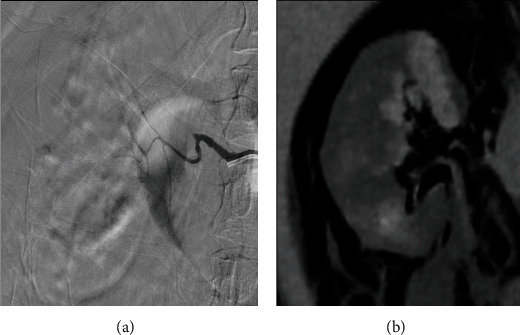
Digital subtraction angiography of the right renal artery (a) demonstrates embolization of the bleeding vessel with no residual bleeding. Coronal venous phase CT image (b) shows a decrease in the size of the subcapsular hematoma and absence of bleeding foci.
